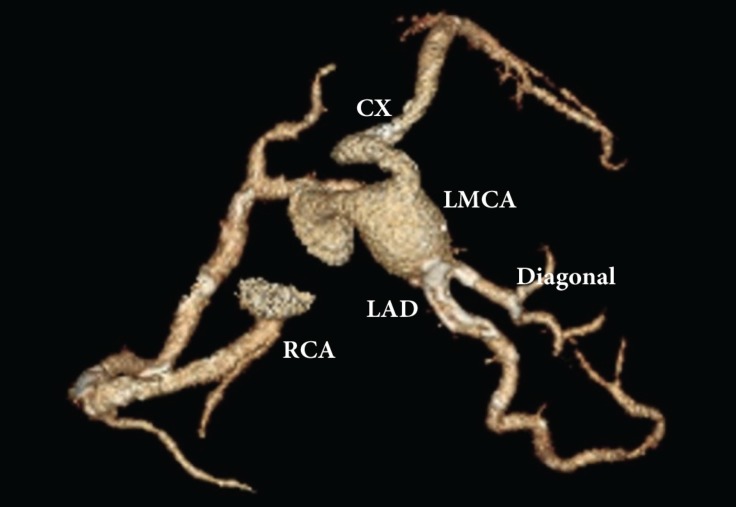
Another view of the giant aneurysm with three-dimensional volume-rendering computed tomography.
